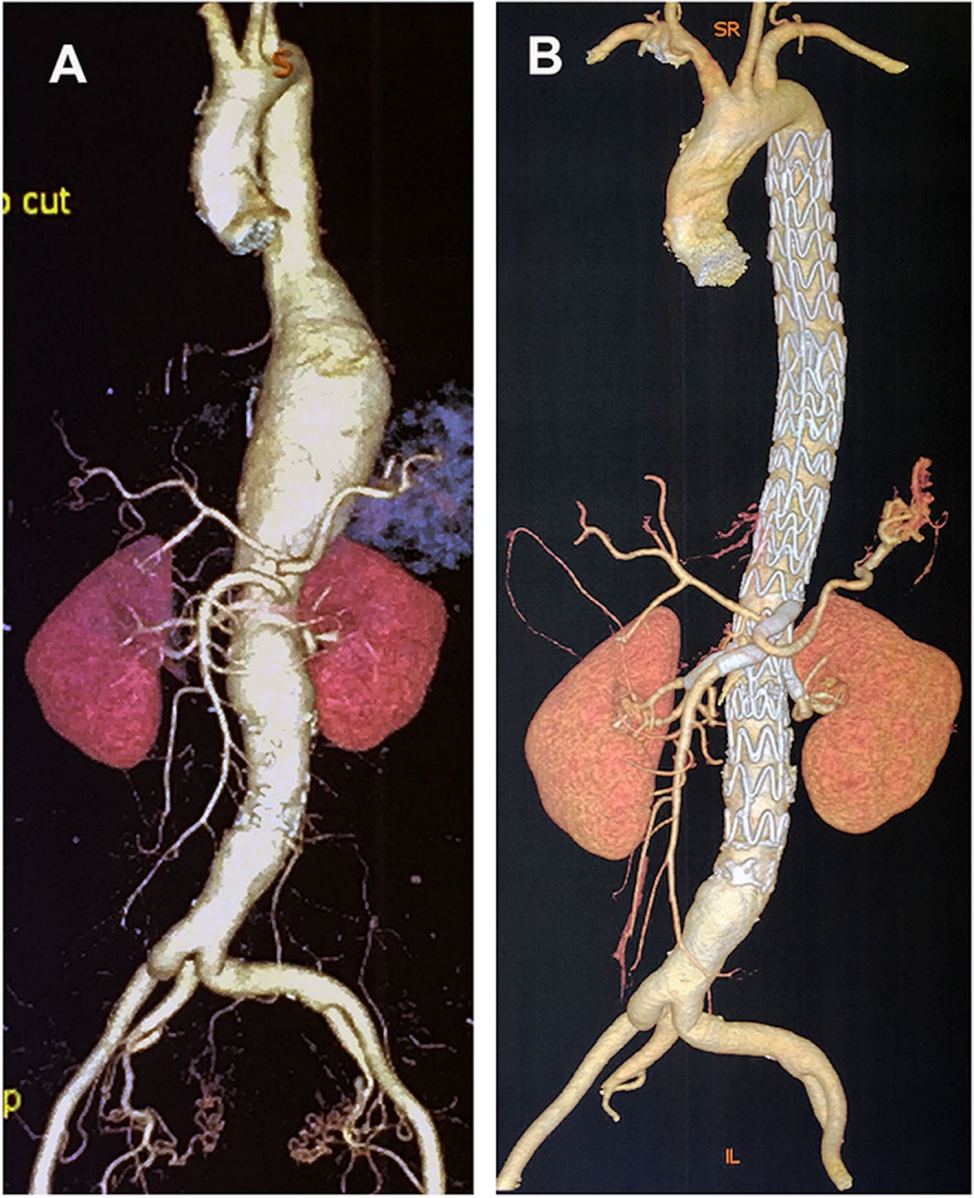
Pre- and postoperative image of the extent III thoracoabdominal aortic aneurysm (TAAA). A Coronal view of the preoperative extent III TAAA. B Postoperative CTA examination in one-month follow-up confirmed patency of all four branches with no endoleak
